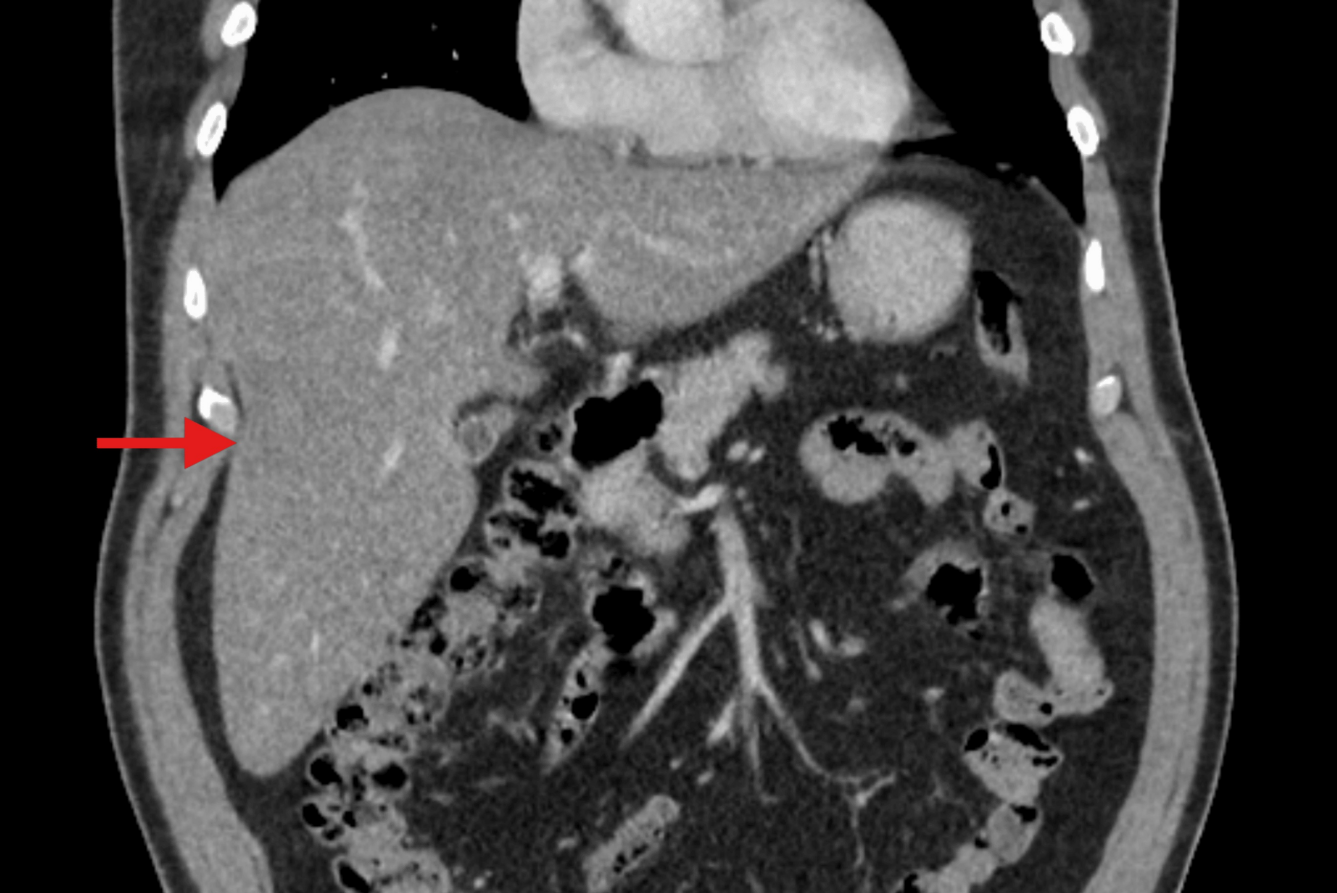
CT abdomen and pelvis with contrast Hepatomegaly with elongated right hepatic lobe measuring 23 cm in the craniocaudal axis (red arrow).
